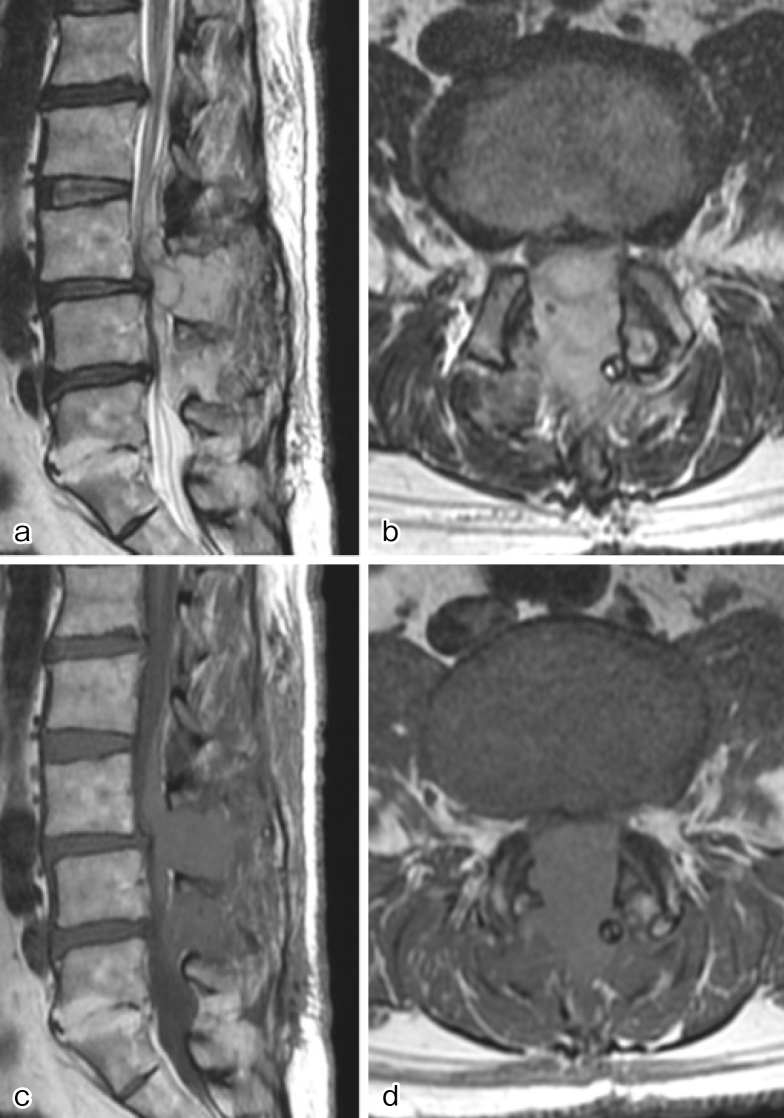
Several hours after the initial surgery, magnetic resonance imaging (MRI) was performed to investigate the cause of bilateral lower limb paralysis and pain. MRI revealed the presence of an epidural hematoma. (a) Sagittal and (b) horizontal sections of T2-weighted images, and (c) sagittal and (d) horizontal sections of T1-weighted images.
